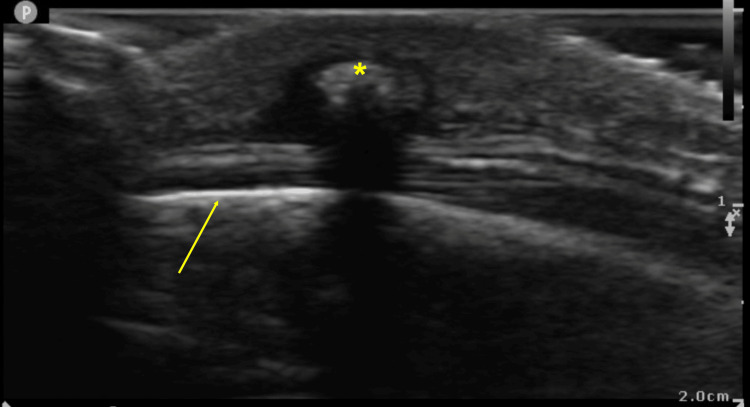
Point-of-care ultrasound findings of right temporal scalp lesion Hyperechoic foreign body (*) superficial to skull (arrow) with surrounding anechoic ring, edema, and posterior acoustic shadowing.
